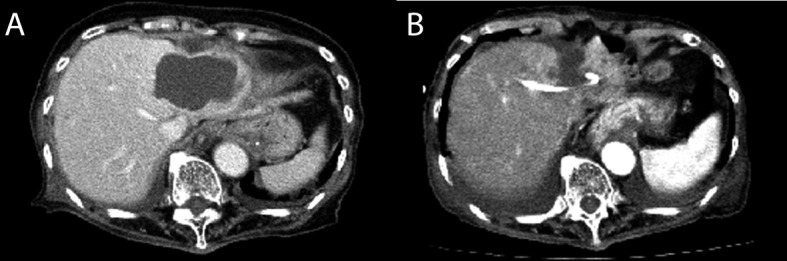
Enhanced liver computed tomography scan on (A) admission and (B) the 3rd day, after the initiation of percutaneous abscess drainage.
